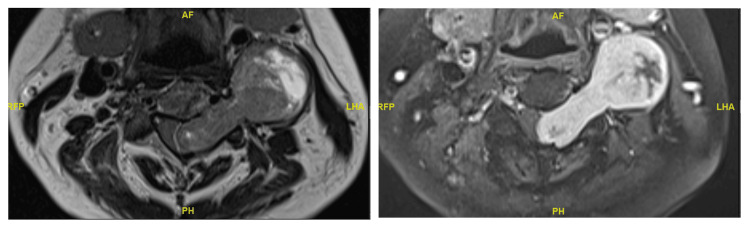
Pre-operative axial MRI images of intradural extramedullary lesion at C3-4 level.
